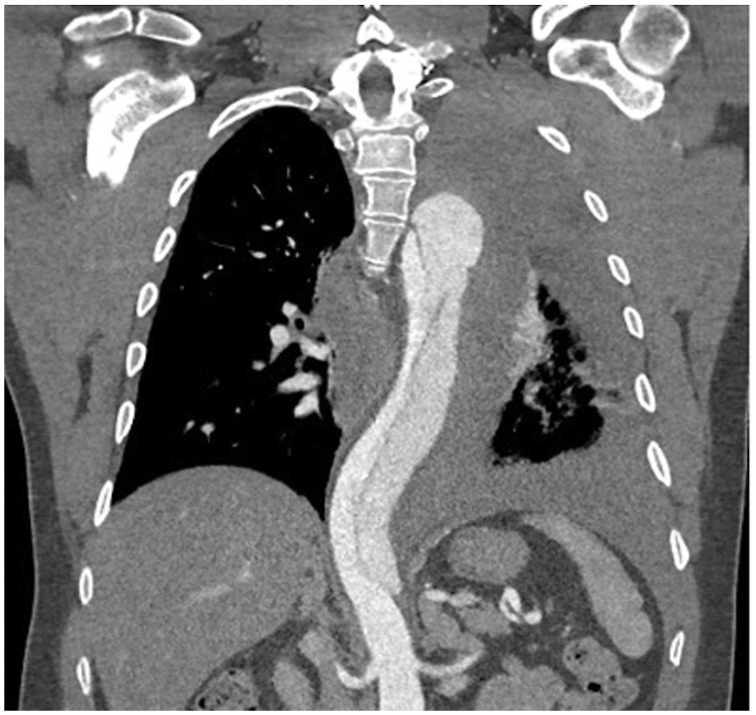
CT aortography showing complex type B aortic dissection.
